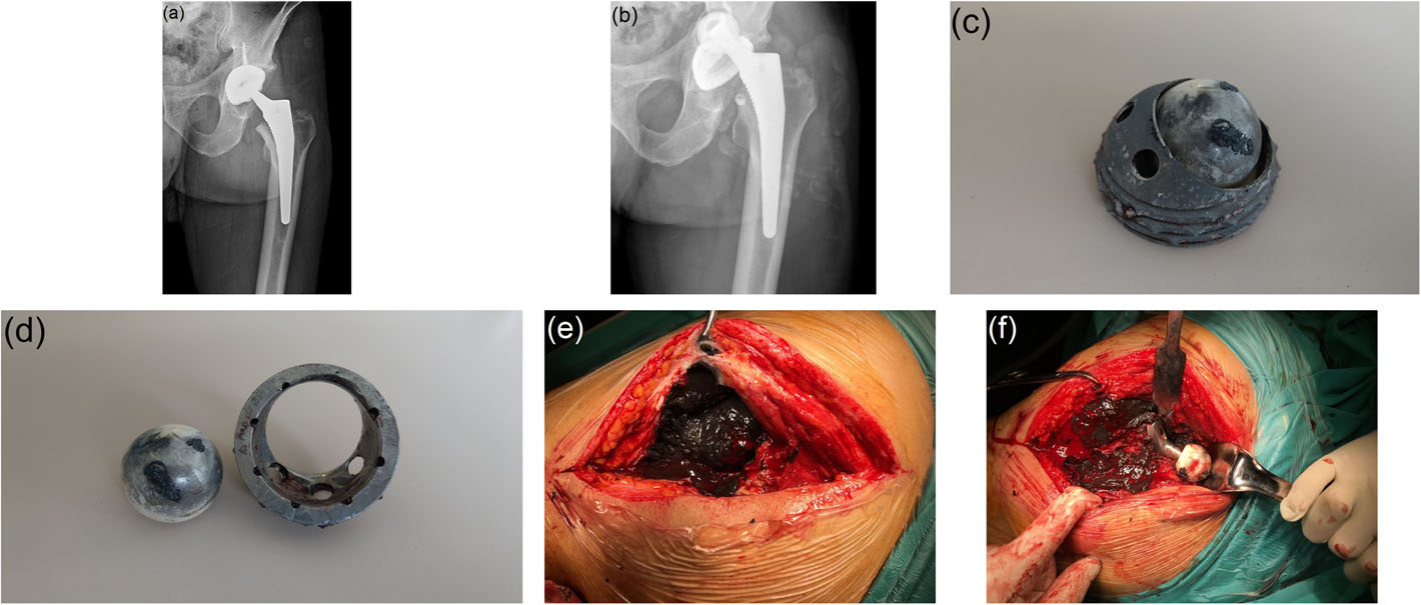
a Anterior-posterior (AP) radiographic image of a 60-year-old man with ceramic-on-ceramic bearing in the 1st year after primary total hip arthroplasty. b AP radiograph 4 years postoperatively showing penetration of the titanium acetabular cup of the ceramic head with complete wear of the ceramic liner. c, d View of the catastrophic deformation of acetabular components. e, f Intraoperative appearance of diffuse metallosis developing in periprosthetic tissues
